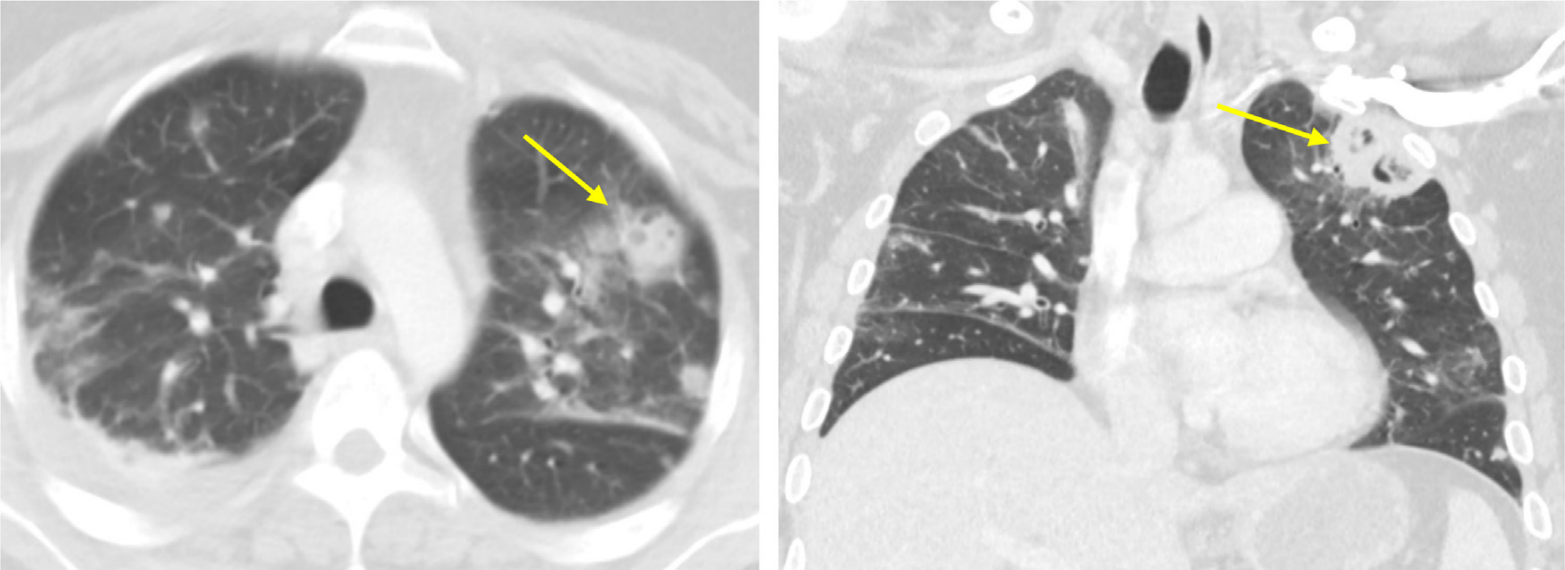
Axial (A) and coronal (B) images of CT scan demonstrate numerous areas of cavitation. Several of the nodules are new and some have demonstrated interval enlargement (yellow arrow) when compared to the prior study (Fig. 1). The large cavitary nodule in the left upper lobe measures 3.0 × 3.1 cm.
